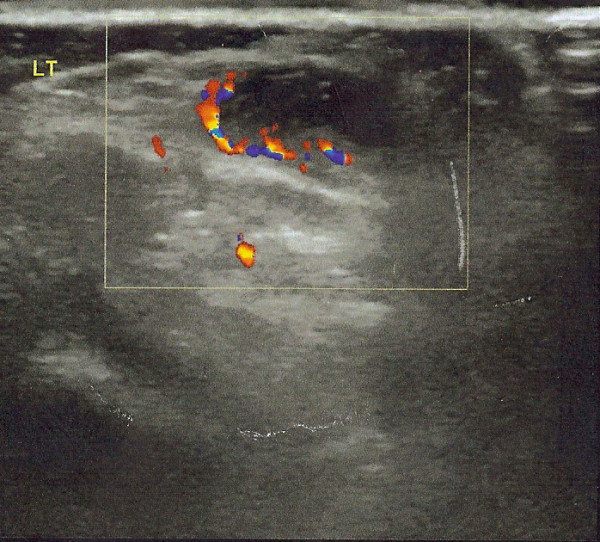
Case 2- A Doppler Sonography demonstrating the blood flow in the mass.
